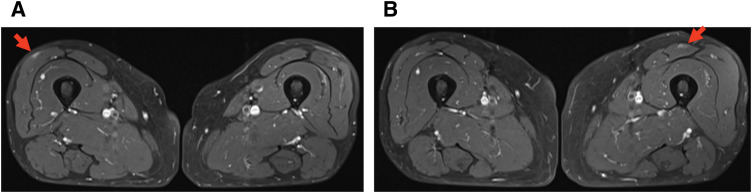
(A) Contrast-enhanced MRI showing a nodule with uniform enhancement in the right vastus lateralis muscle (red arrow). (B) Contrast-enhanced MRI showing a nodule with uniform enhancement in the left rectus femoris muscle (red arrow).
